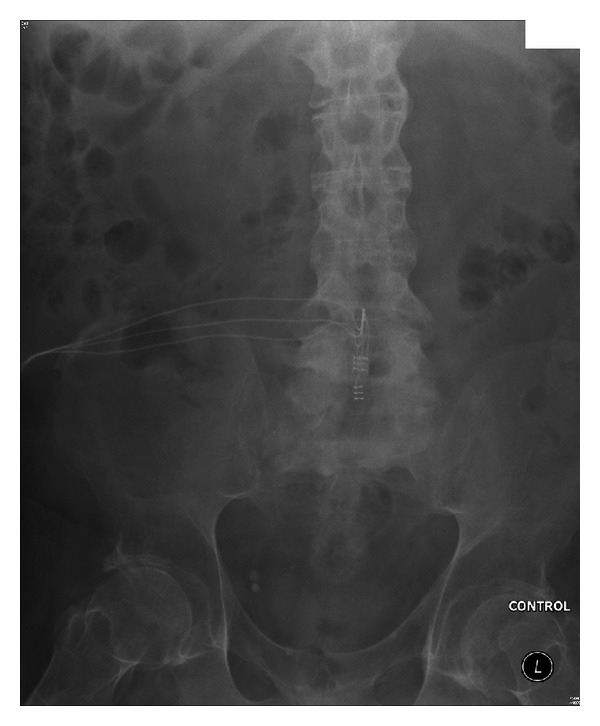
Case 3: X-ray kidneys and urinary bladder, taken in September 2009, showing no radioopaque urinary calculi. The receiver block which had been implanted in the right flank had been removed because of *Staphylococcus aureus* infection.
